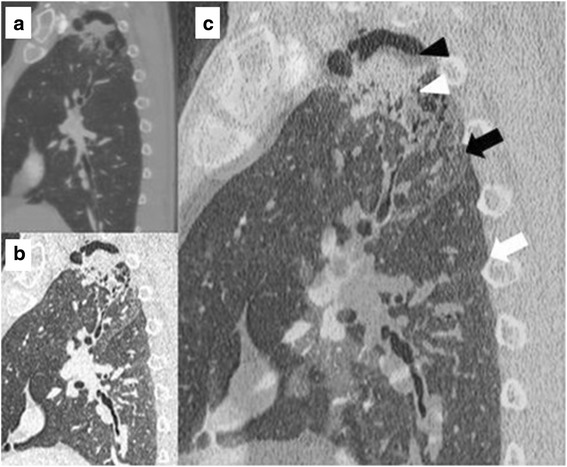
Images of a case of partial strong pleural adhesion (adhesion grade 2). a Sagittal CT image of inspiration phase. b Sagittal CT image of expiration phase. c Two images superimposed. Tumor was fixed during two phases of inspiration. White arrow indicates fissure line in inspiration phase. White arrow head indicates a tumor in inspiration phase. Black arrow indicates fissure line in expiration phase. Black arrow head indicates a tumor in expiration phase
